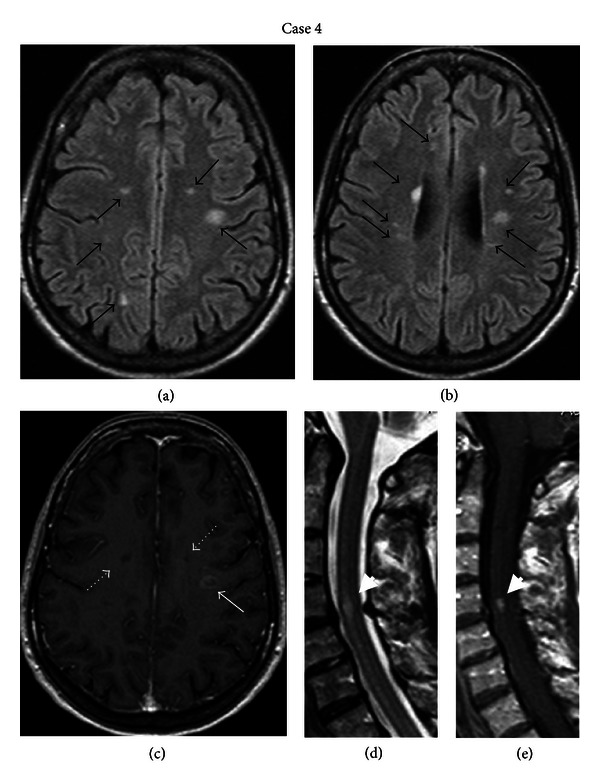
(a) and (b) Axial FLAIR brain MRI images of the fourth patient demonstrating multiple periventricular and subcortical lesions at the frontal, parietal, and temporal lobes (black arrows). (c) Axial T1-weighted brain MRI image showing gadolinium ring enhancement in one of the subcortical lesions (white arrow) and two subcortical black holes (white dotted arrows) (e) Sagittal T2- and (f) T1-weighted MRI of the cervical spine showing one hyperintense lesion at the C4-C5 level with gadolinium enhancement (white arrowheads).
